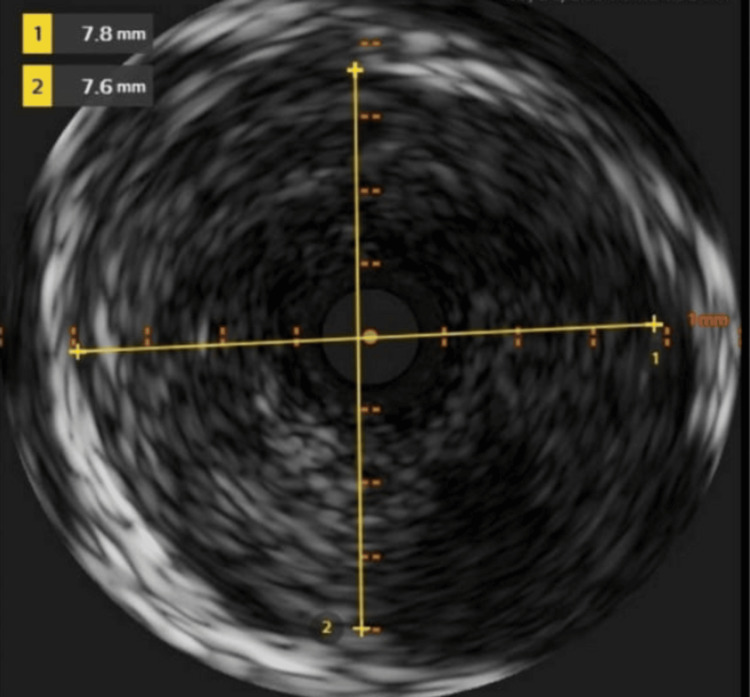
Intravascular ultrasound (IVUS) still frame showing the ectatic segment, with inner luminal diameters measuring 7.8 × 7.6 mm.
